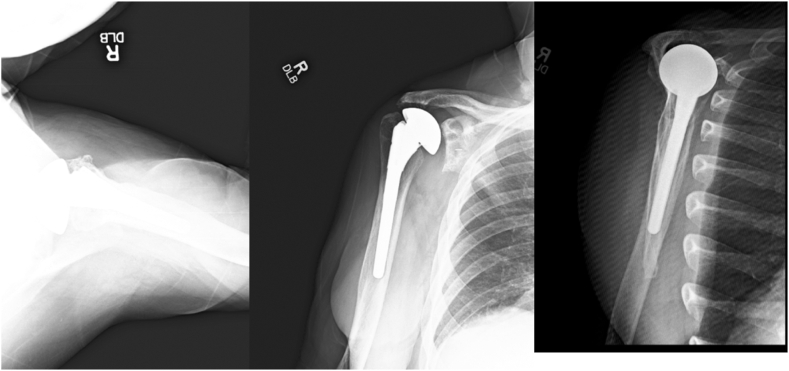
Radiographs revealing the original anatomic shoulder arthroplasty with in place TSA with lucencies around the glenoid component, indicating glenoid loosening. There is also superior migration of the humerus. *TSA*, total shoulder arthroplasty.
